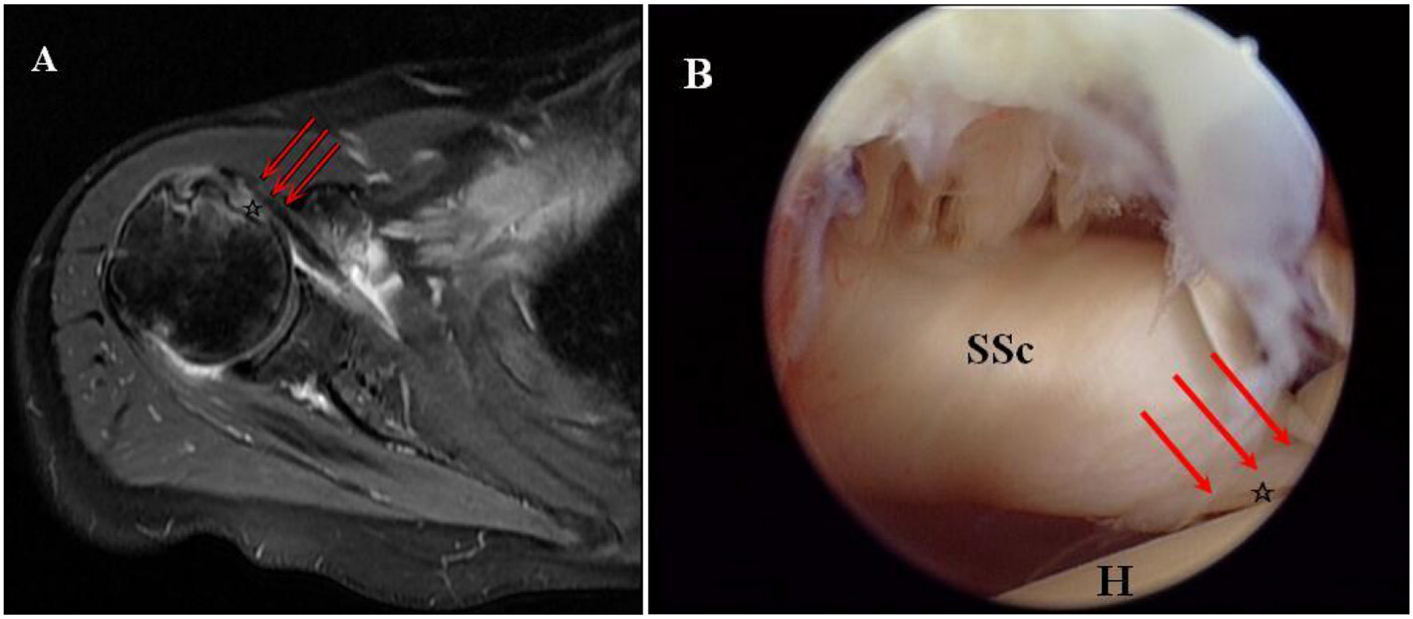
Torn subscapularis tendon without retraction in the right shoulder of a female patient aged 64 years. (A) Torn subscapularis tendon (asterisk) without retraction showed on preoperative axial T2-weighted MRI. (B) Non-retraction of the torn subscapularis tendon (asterisk) was detected according to an arthroscopic intra-articular view from the posterior portal (SSc, subscapularis; H, humeral head).
